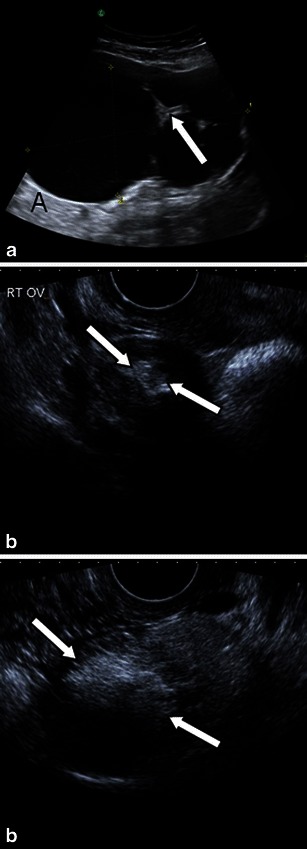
Difference in ultrasound features between struma ovarii and ovarian dermoid cyst. a Predominantly cystic struma ovarii typically mimicking an epithelial ovarian tumour (either cystadenoma or borderline ovarian tumour) in the right ovary of a 51-year-old patient presenting with non-specific abdominal cramps. Some thicker septations are seen (*arrow*) between otherwise cystic areas presenting with anechoic fluid and posterior acoustic enhancement (*A*).*Right* 1.5-cm (b) and *left* (c) 5.1-cm dermoid cyst in a 24-year-old woman. Both lesions on ultrasound have the typical pattern of high attenuation within the cyst (*arrows*) representing its fatty component
